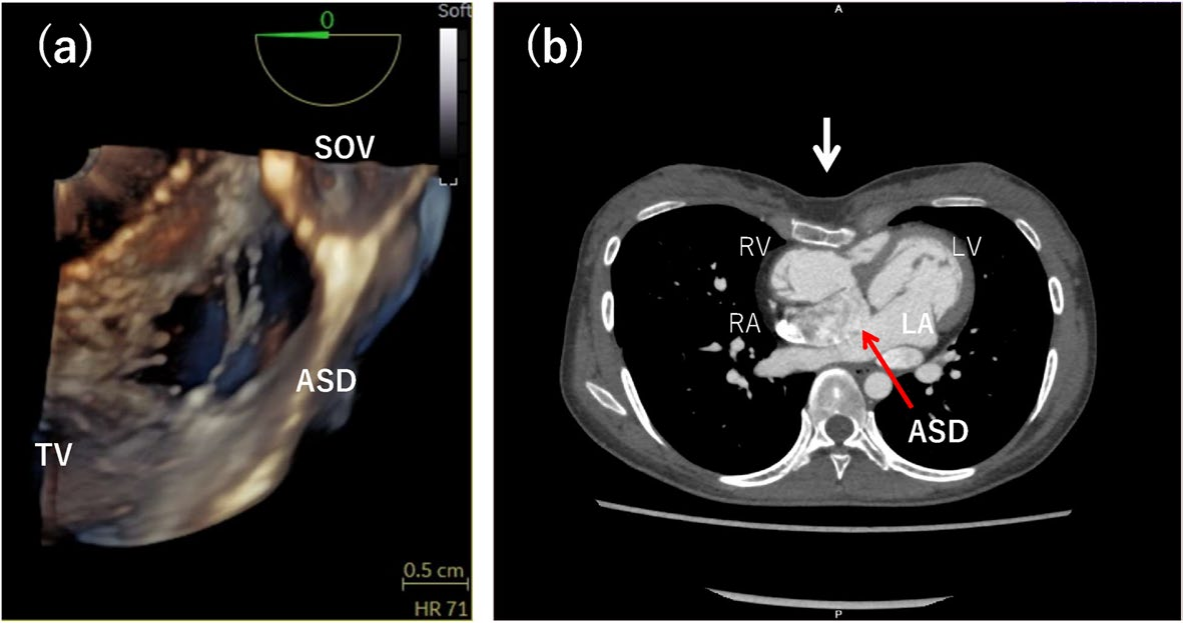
Imaging findings. a Three-dimensional transesophageal echocardiography (TEE) from the right atrial side showing an atrial septal defect (ASD) divided into three orifices by two septal structures. b Chest computed tomography showing an ASD (red arrow) and right ventricular compression due to sternal depression (white arrow). ASD, atrial septal defect; TV, tricuspid valve; SOV, sinus of Valsalva; RV, right ventricle; RA, right atrium; LV, left ventricle; LA, left atrium
